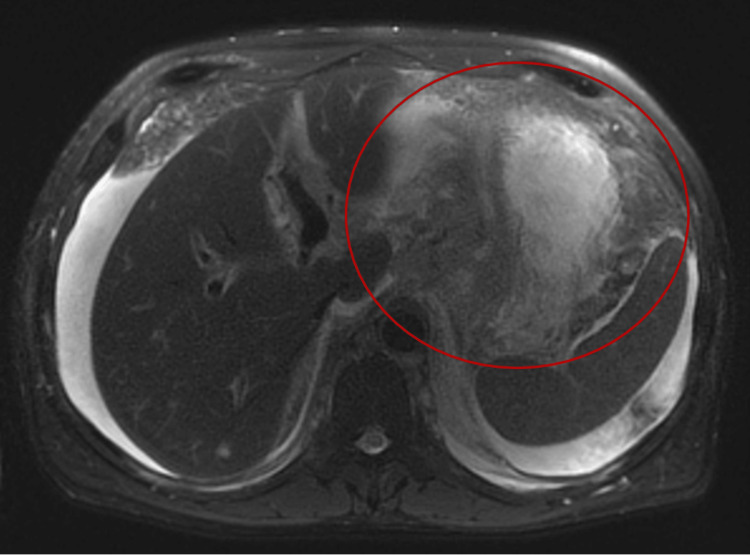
MRI of the abdomen demonstrating a large, heterogeneously enhancing mass involving the gallbladder and adjacent liver parenchyma (red circle). Differential considerations include gallbladder carcinoma and cholangiocarcinoma.
